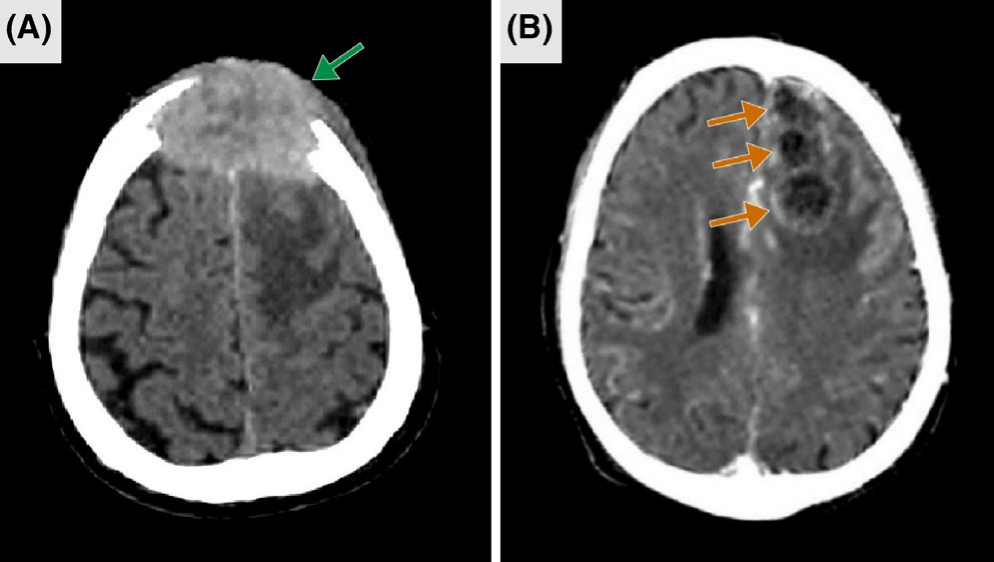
Brain computed tomography (CT). Axial CT scan without contrast reveals a frontal osteolytic lesion measuring 35 × 59 × 53 mm (green arrow) (A). Axial CT scan with contrast shows three ring‐enhancing lesions in the left frontoparietal area (orange arrows) (B)
